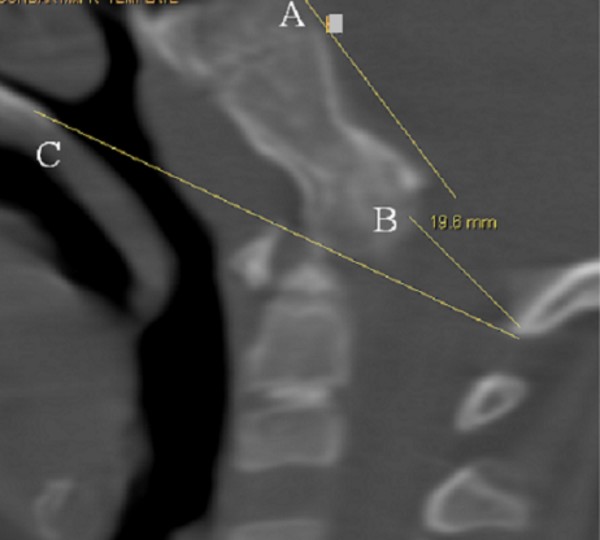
3 D sagittal CT scan showed a hypertrophied clivus. Line (A) is the Wachenheim clivus line (a method to evaluate and assess craniocervical junction abnormality), for which a line drawn along the posterior aspect of the clivus toward the odontoid process. In normal individuals the line must intersect/ tangential to the odontoid. In our present patient the line is remarkably deviating, outlining the existence of significant craniocervical abnormality. Line (B) is McRae’s line, which is essentially a measurement across the foramen magnum, and is drawn from the tip of clivus (basion) to opisthion (posterior margin of foramen magnum). McRae’s line is generally longer than 30 mm in normal individuals. A diameter of less than 25 mm is almost always associated with neurological abnormalities. In our patient McRae’s line showed a length of 19.6 mm. Line (C) is McGregor’s line, which is drawn from the posterior edge of the hard palate to the most caudal point of the occipital curve. An odontoid tip extending more than 4.5 mm above this line is considered abnormal. In our patient the hyperplastic clivus (the basion) is reversing the mechanism by extending downwards and joining the cephalad part of the odontoid.
